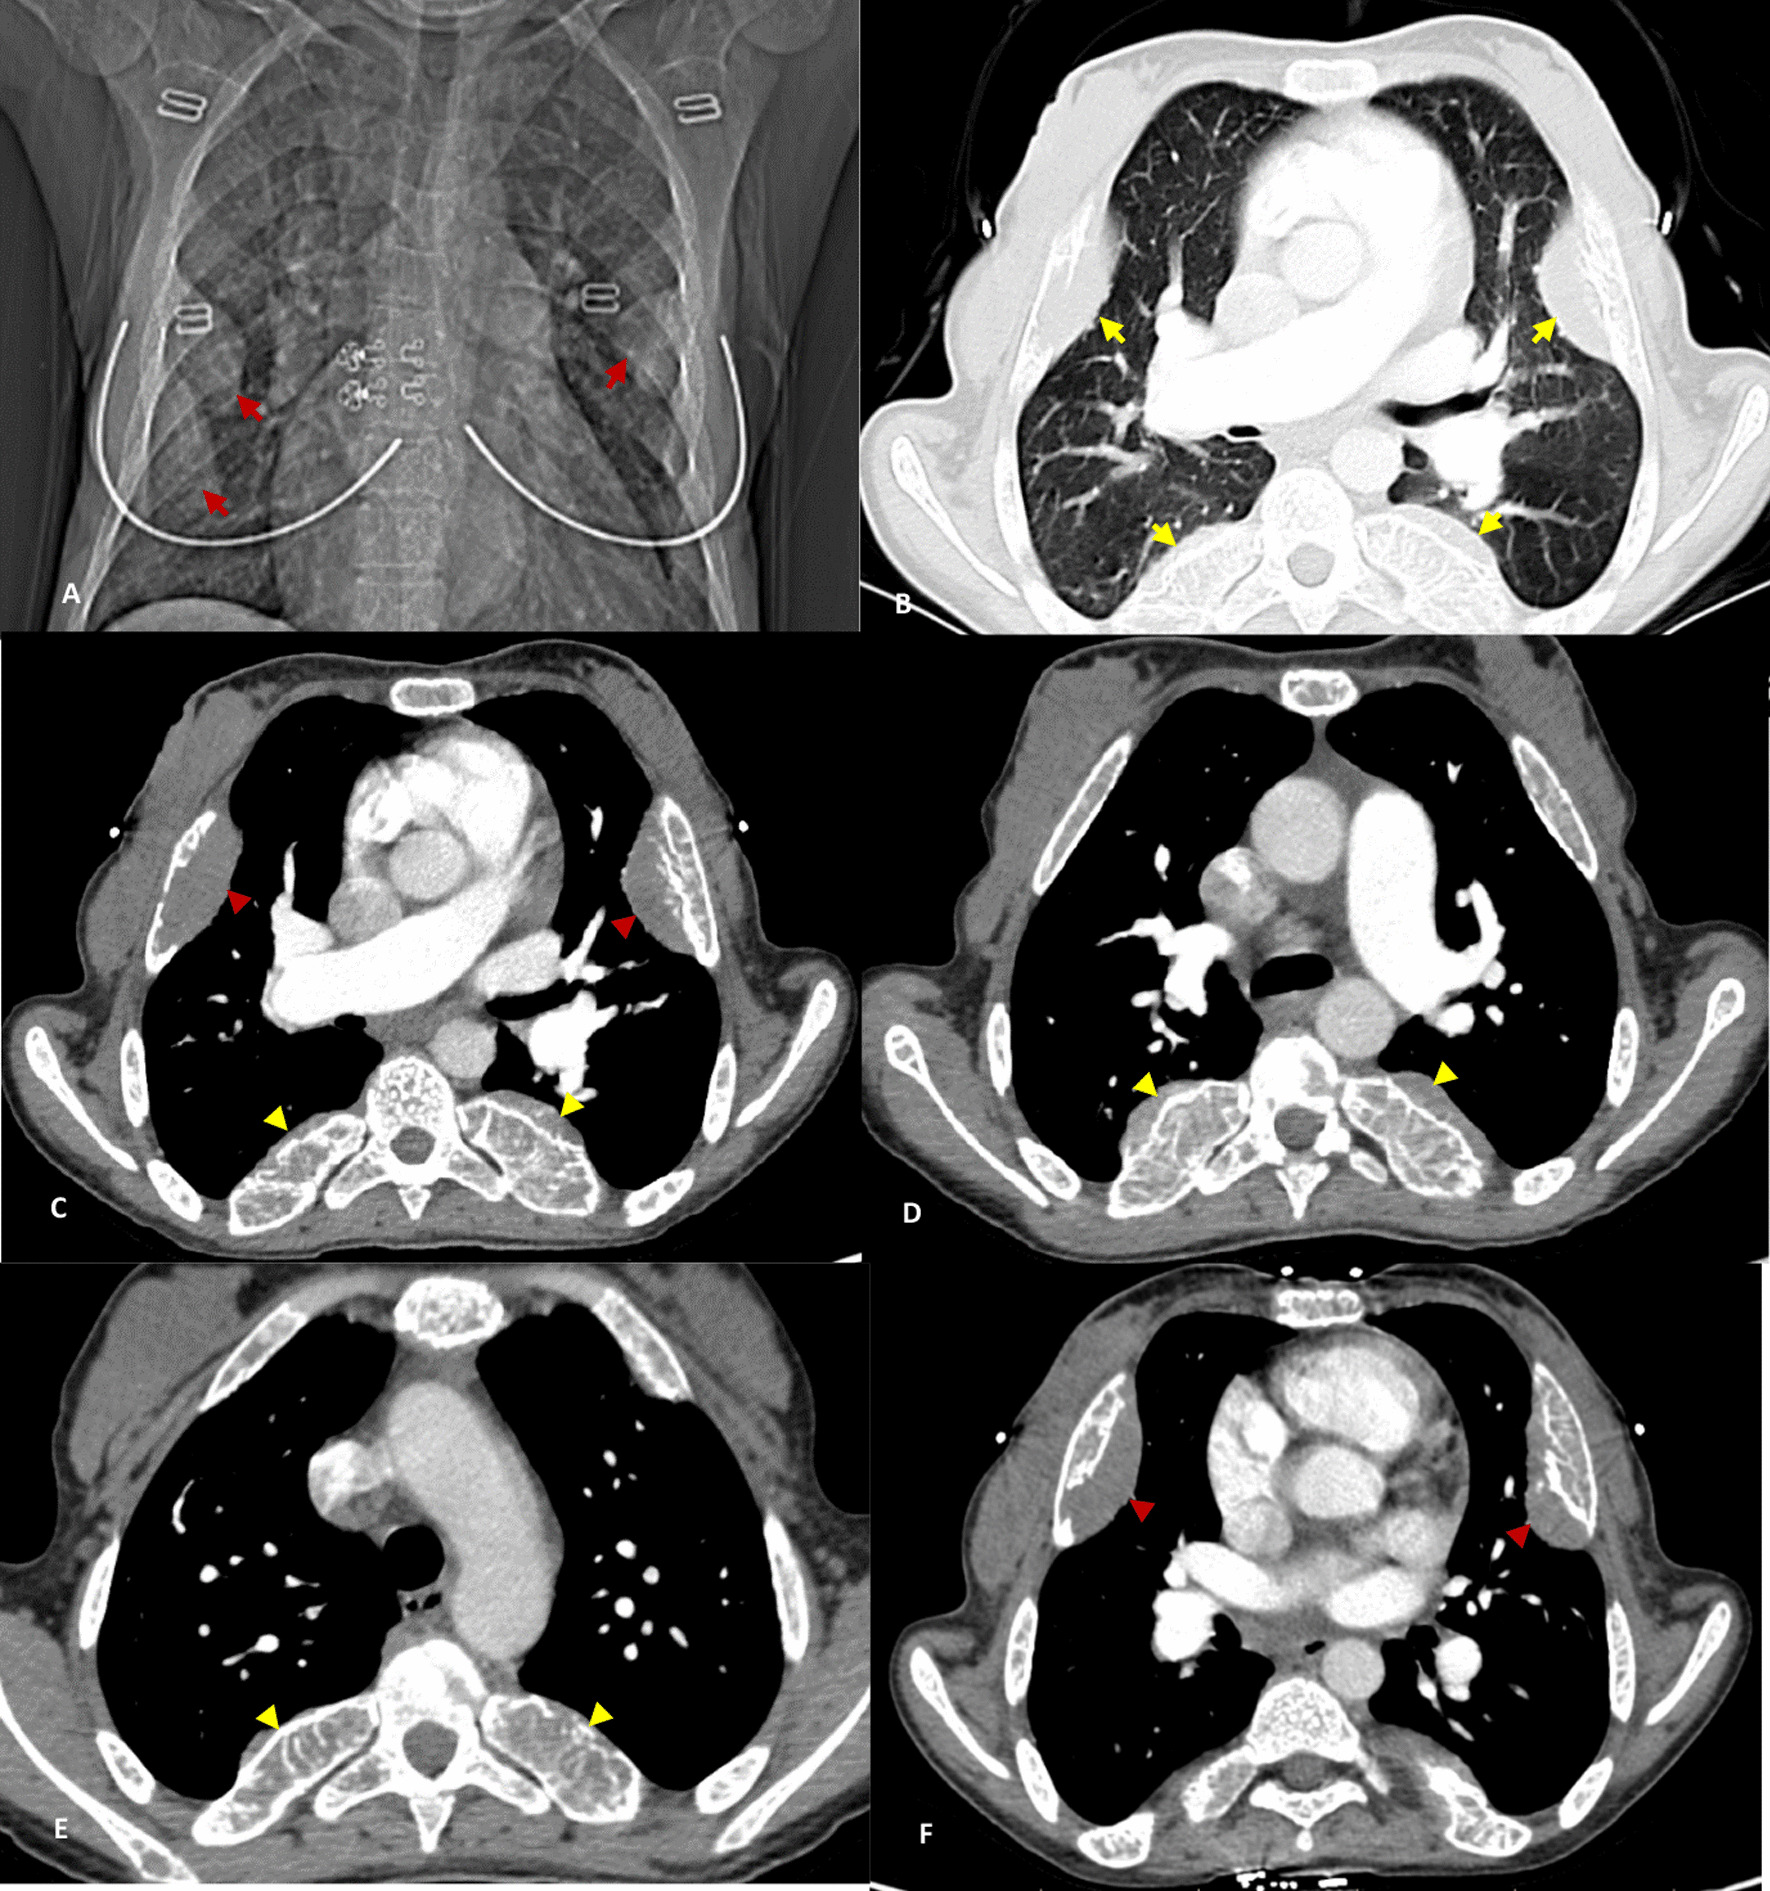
A Plain radiography with rib lesions (red arrows). B Parenchymal view on computed tomography scan with multiple rib lesions (yellow arrows). C–F Bilateral rib lesions in lateral (red arrowhead) and posterior sections of ribs (yellow arrowhead)
